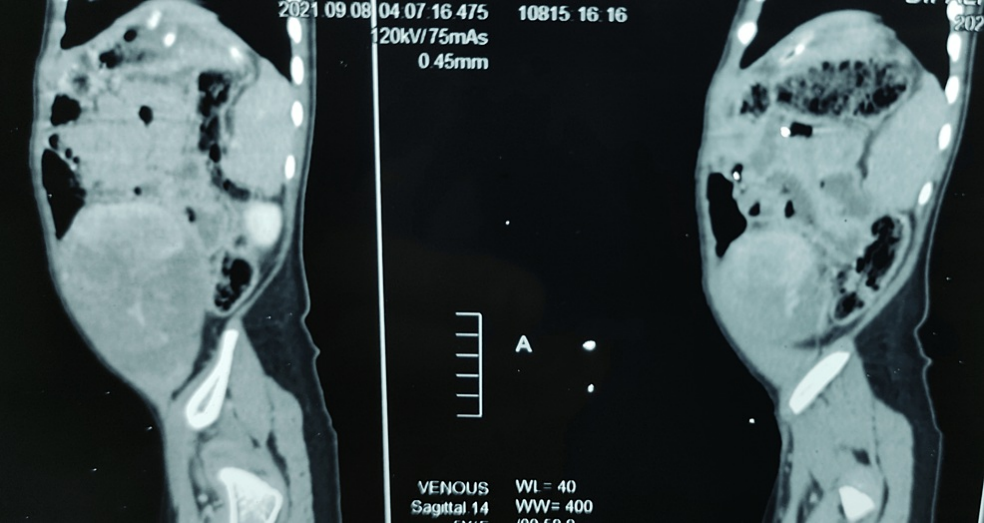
CECT abdomen (sagittal section) CECT: Contrast-enhanced computed tomography
